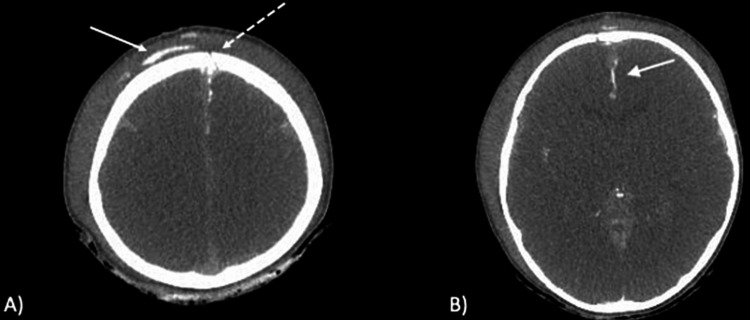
Head CT image shows (A) an extravascular leakage image (white arrow) outside the skull. The extravascular leak is continuous from the intracranial area through the skull fracture (dotted arrow). (B) extravascular leak (white arrow) in the frontal longitudinal of the cerebrum, suggesting that the cause of the hemorrhage may be the ACA.
